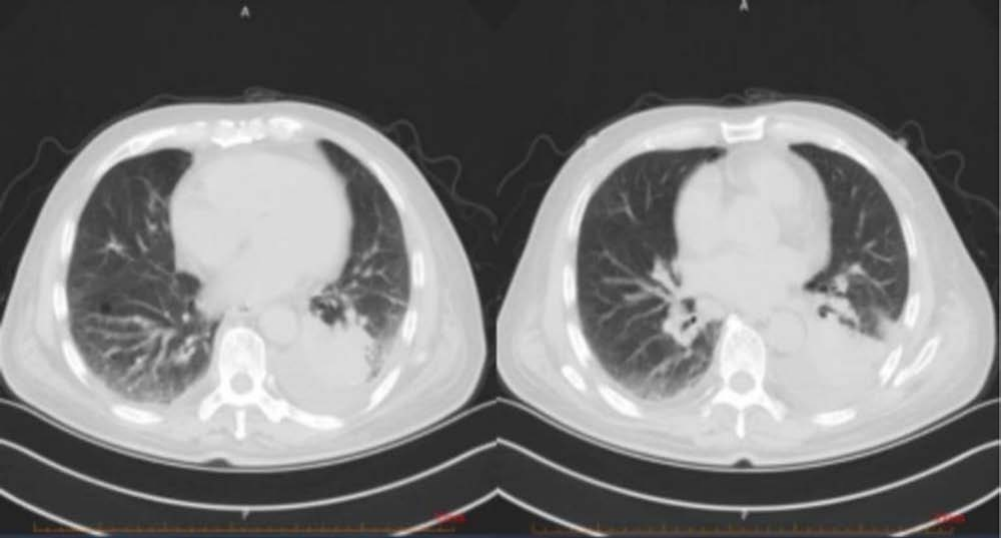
Lung CT on March 11th: compared with March 4th, the lung field transparency has increased significantly, and the exudative lesions in both lungs have become more severe. The range of patchy high-density shadows in the lower lobe of the left lung has slightly decreased compared to before. CT = computed tomography.
